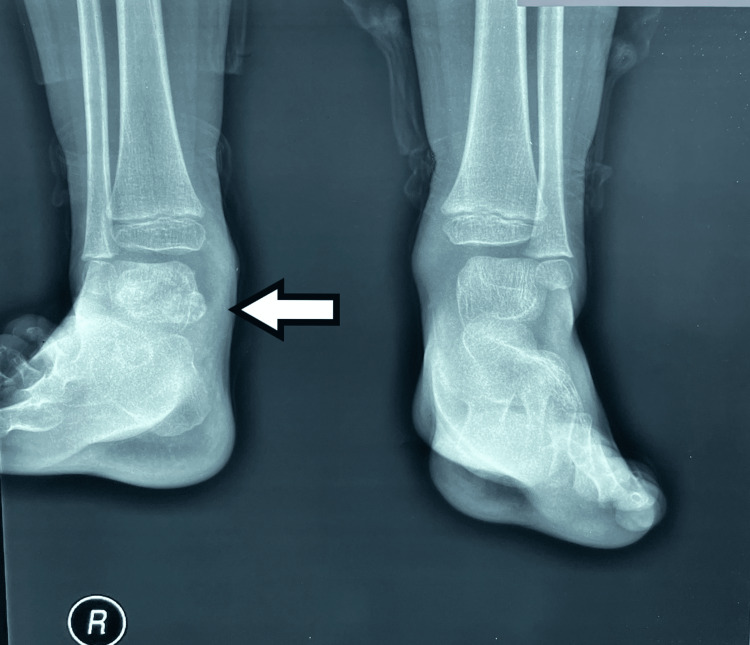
Plain radiographs of the ankle joints in anteroposterior projection. A large calcified lesion, with lobulated margins, is observed adjacent to the posterior margin of the right talus.
